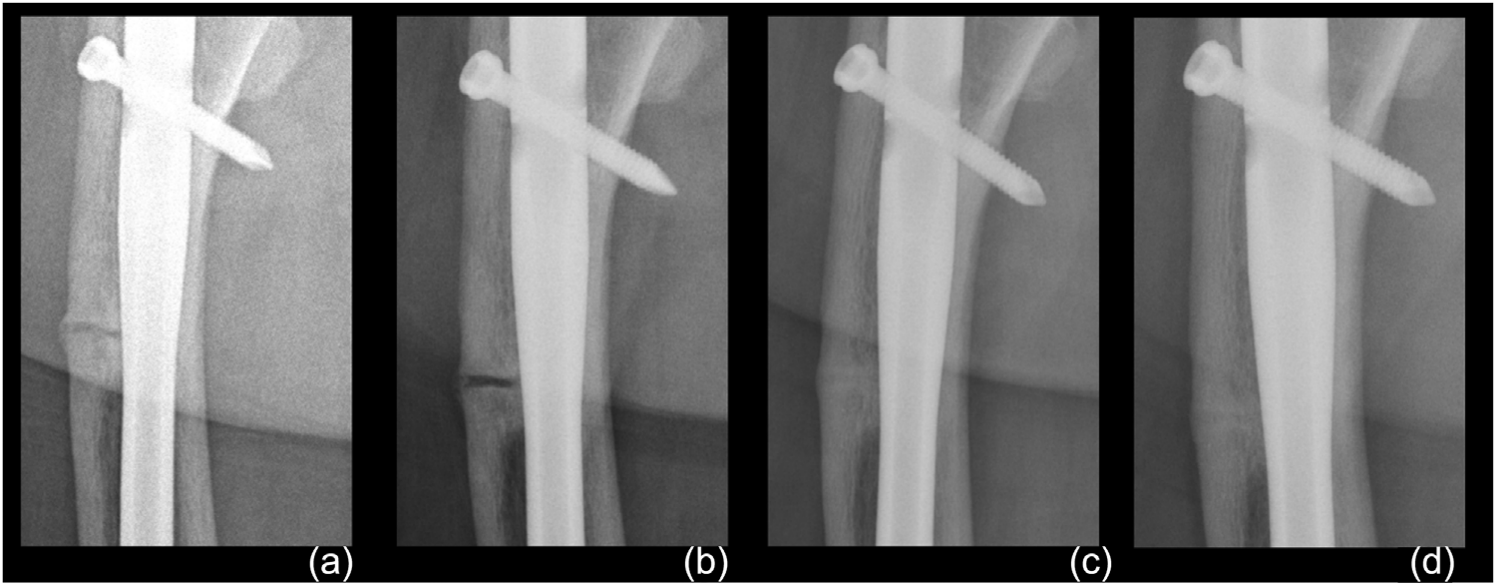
Case 1: Radiograph series of right subtrochanteric femoral pseudofracture. (*A*) Right femur pseudofracture 12 years after identification; before asfotase alfa treatment. (*B*) Stable 17 years after fracture; 1 month before asfotase alfa therapy. (*C*) Eleven months of uninterrupted asfotase alfa treatment; radiograph demonstrates progressive healing of femoral pseudofracture. (*D*) Fourteen months on medication; continued bone healing.
